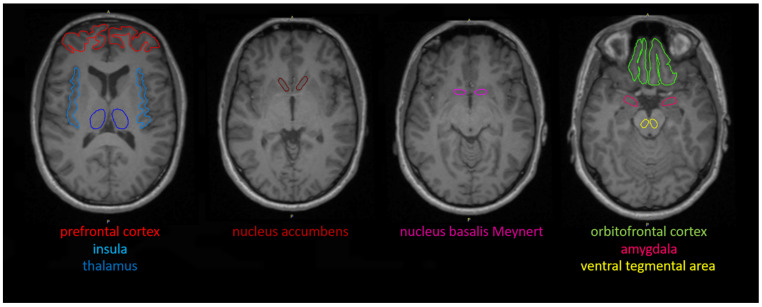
Manually drawn, anatomical-based volumes of interest defined in individual transaxial magnetic resonance imaging data sets (same text color was used for the specific brain region in each slice).
